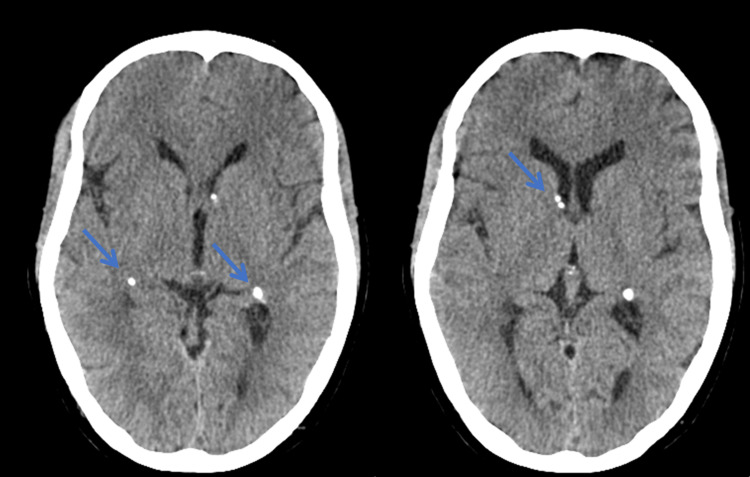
Cranioencephalic CT. Subependymal, non-specific gross calcifications along the walls of the lateral ventricles and predominant right parietal subcortical hypodensities (blue arrow). CT: computed tomography
